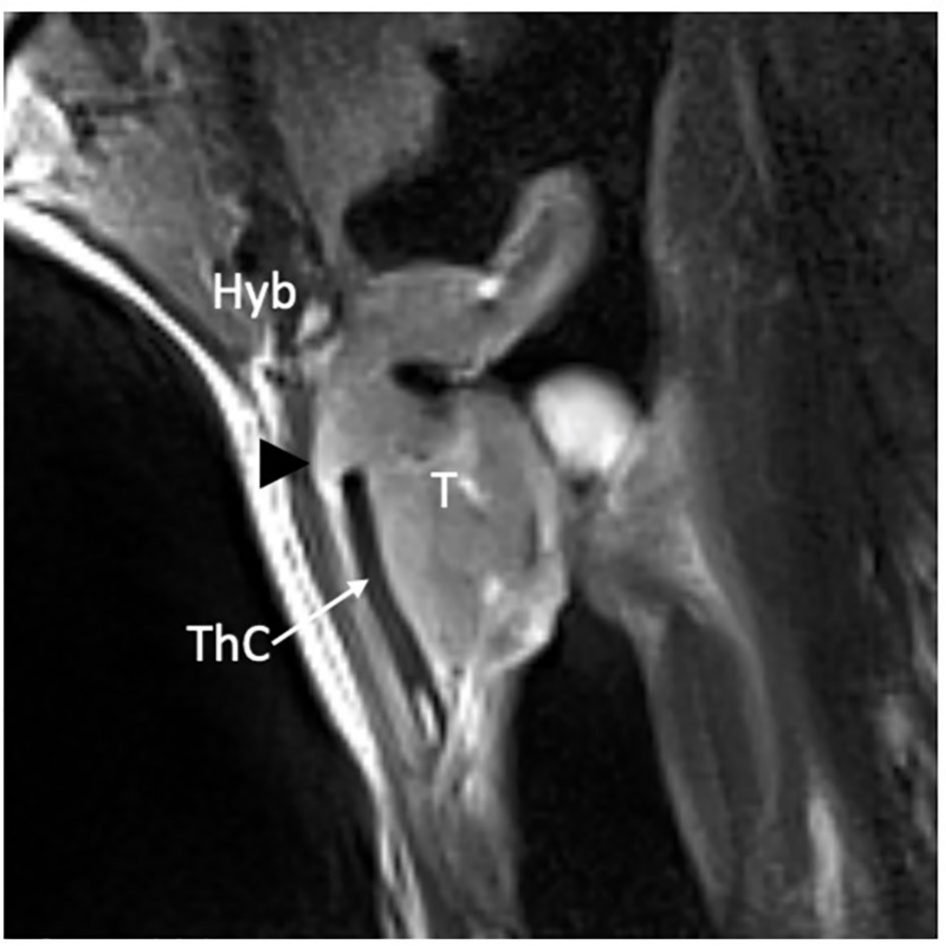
MRI T2-weighted image on parasagittal plane demonstrates a supraglottic lesion (T) that reaches the thyroid notch (black arrowhead) with suspected infiltration of the thyrohyoid membrane above the superior margin of a non-ossified thyroid lamina (ThC) that shows regular signal intensity as the hyoid bone (Hyb).
